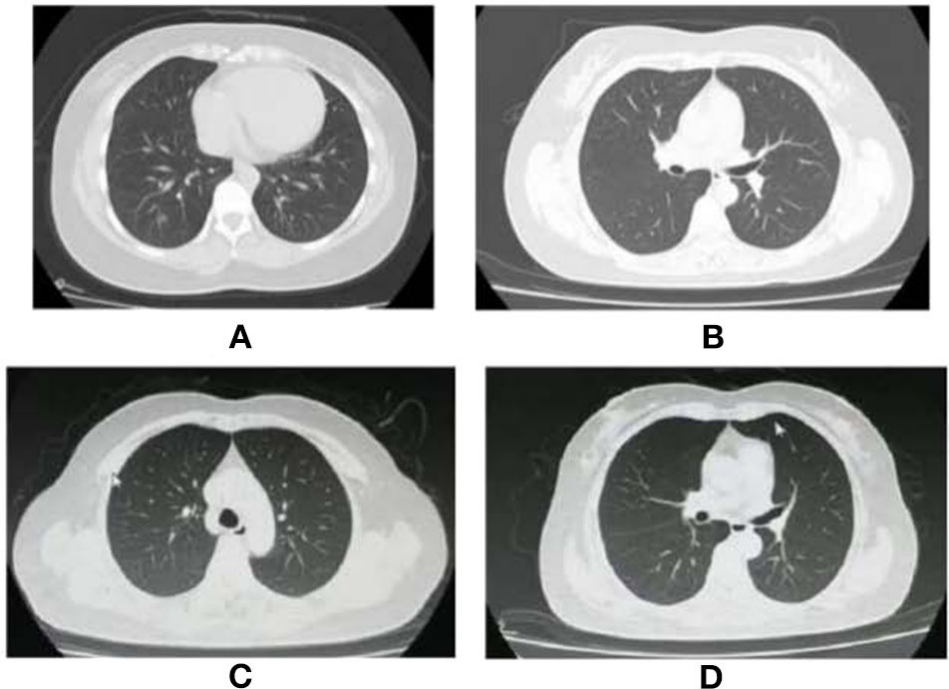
Chest CT images of two cases with COVID-19. (A) The Chest CT images of the Case 1 showed ground-glass opacities in basal segment of the lower lobe of the right lung on January 2. (B) The Chest CT images of the Case 1 showed almost normal on March 23. (C) The Chest CT images of the Case 2 showed almost normal on April 7. (D) The Chest CT images of the Case 2 showed almost normal on June 21.
